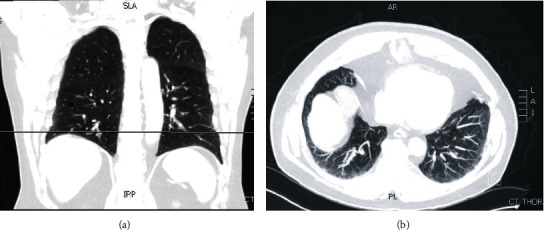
Case 1. (a) CT scan chest coronal view demonstrating subpleural reticulation within the periphery of the lung as well as thin-walled cysts of various sizes (arrows). (b) CT chest using maximum intensity projection protocol demonstrating areas with the most disease activity and least vasculature (circle).
